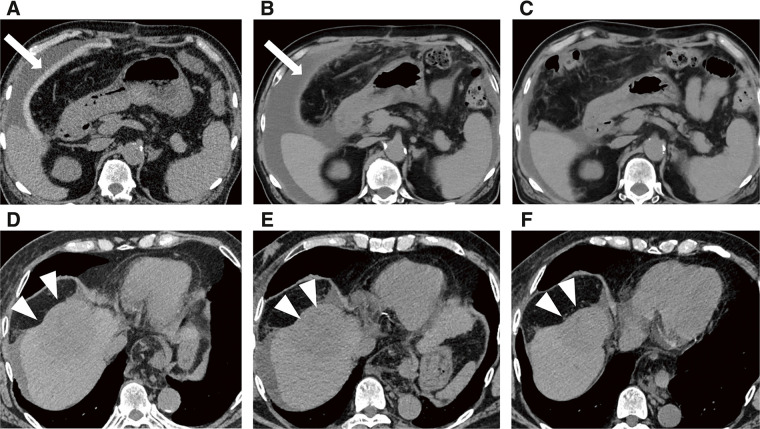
Changes in the abdominal computed tomography of the polyglycolic acid spacer and the tumor after the treatment. Abdominal computed tomography taken at (A) 1 month, (B) 2 months, and (C) 3 months after proton therapy showing that the polyglycolic acid spacer was gradually absorbed and disappeared (white arrows). Abdominal computed tomography taken (D) 3 months, (E) 12 months, and (F) 26 months after proton beam therapy, revealing no apparent sign of recurrences (white arrowheads).
